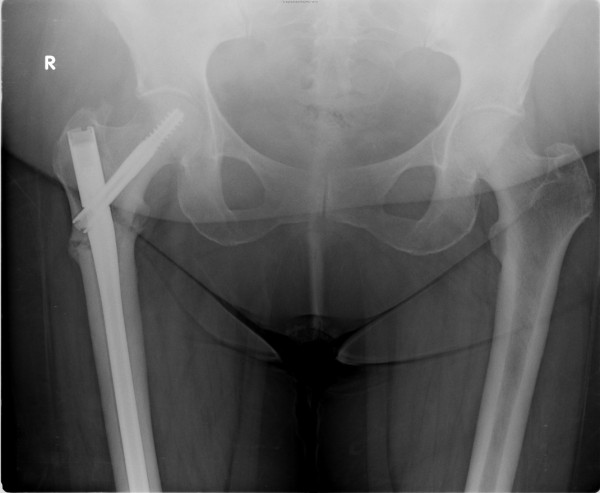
X-ray of the right hip six months following Gamma nail insertion with delayed bone healing process.
